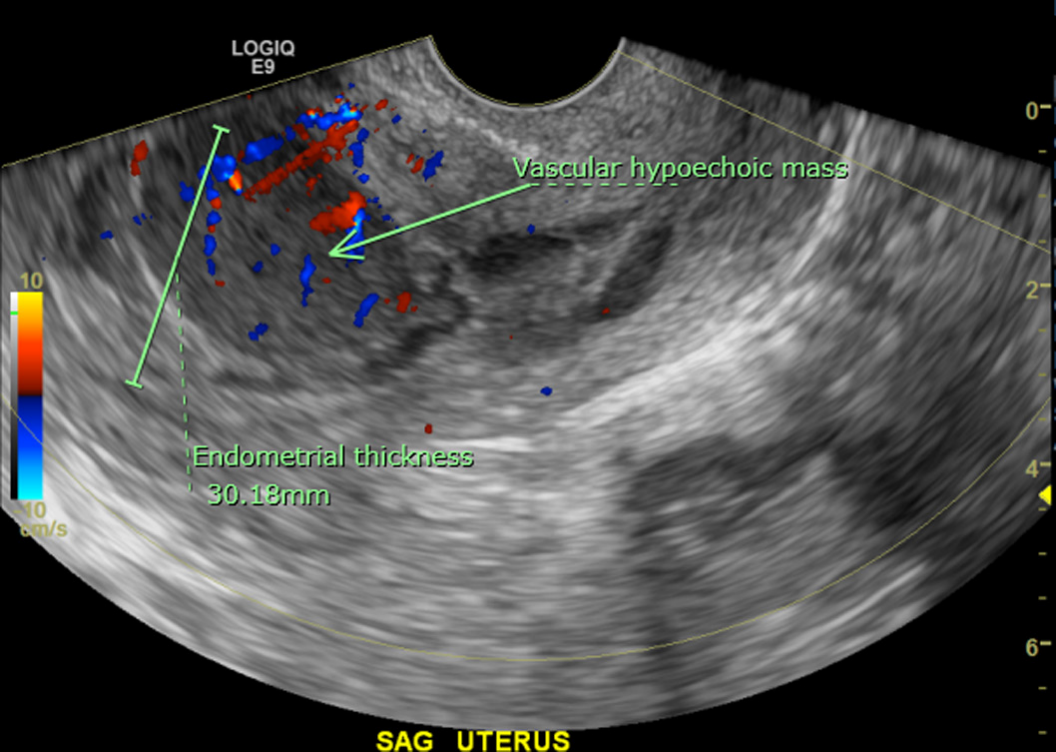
Transvaginal gray‐scale ultrasound image of the uterus in the sagittal plane. The endometrial stripe is markedly thickened to 30 mm with an irregular contour delineated by a small amount of fluid within the endometrial cavity. There is a 3.2 × 2.2 × 3.3 cm^3^ ovoid homogeneous hypoechoic mass within the anterior endometrium with internal color Doppler flow and a low resistance arterial waveform.
